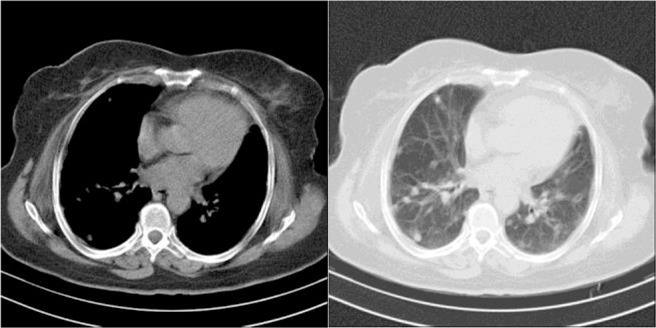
Computed tomogram showing a large solid lobulated margin mass lesion involving the left lung lobe and also the right lung lobe
